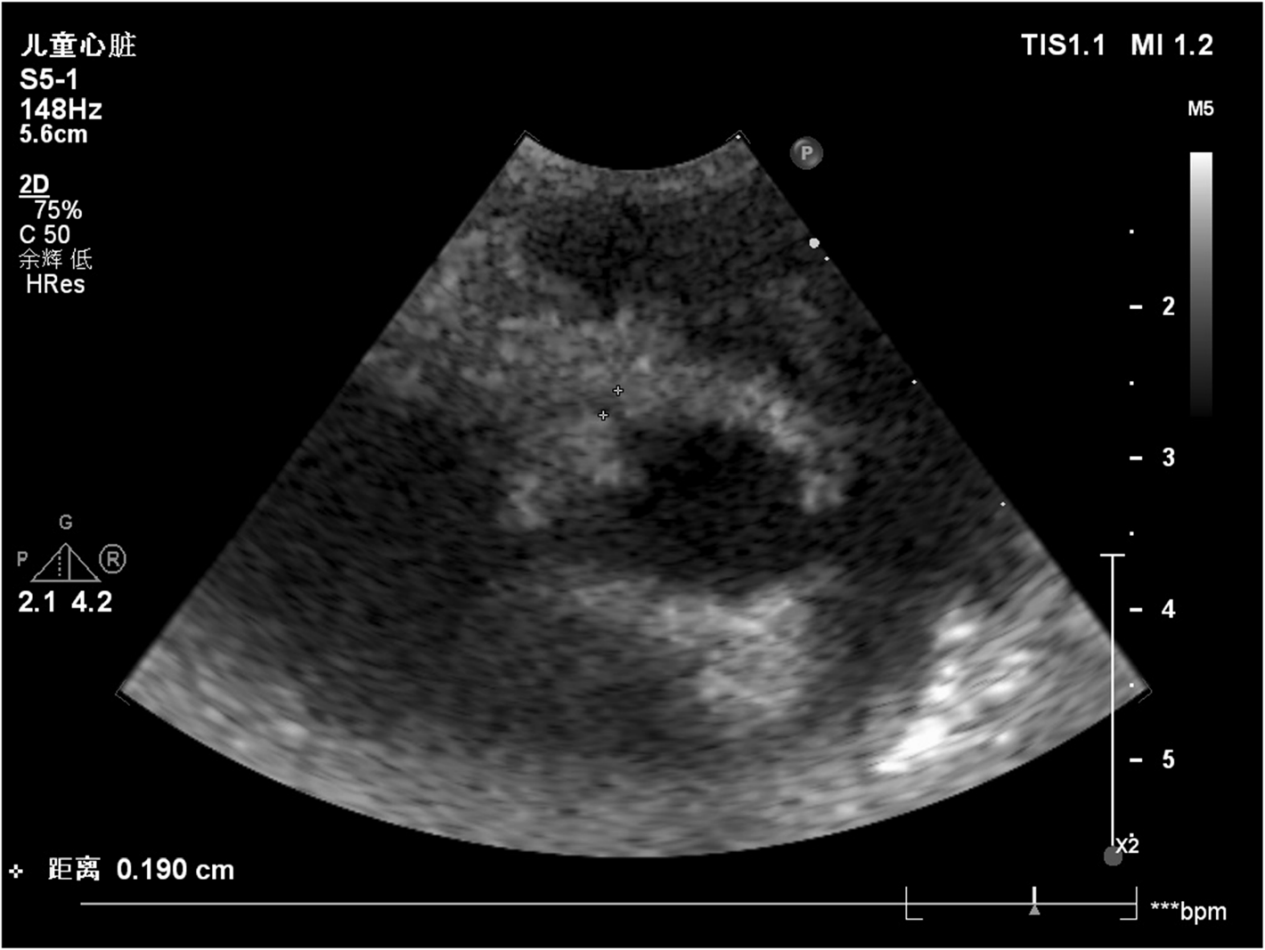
A gradual regression was observed in the CA aneurysm on echocardiography after 1 month
